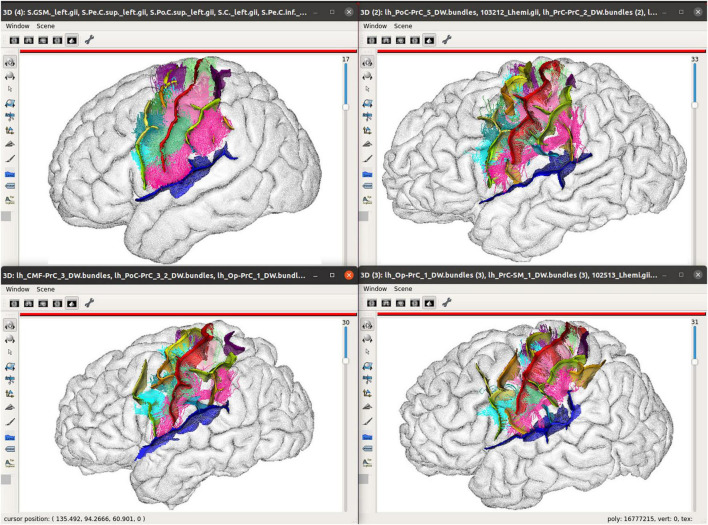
Multimodal structural atlas browsing; A subset of sulcal graphs and labeled fiber bundles are displayed simultaneously for three different subjects and an average model in a selected region of the brain. The top left view displays the sulci computed from the average icbm152 MRI using Morphologist and a few of the short bundles of an 80-subject atlas (Labra et al., 2019). The other views show instances of the sulcus and bundle structural atlases in 3 individual subjects, each in its individual native space. Sulcal and bundles data are displayed with nomenclatures, which are used to identify the same structures across subjects, and assign colors to them. Hemisphere brain meshes are displayed in a wireframe mode, here, to allow a better visualization of the fiber trajectories, which circumvent the folds.
